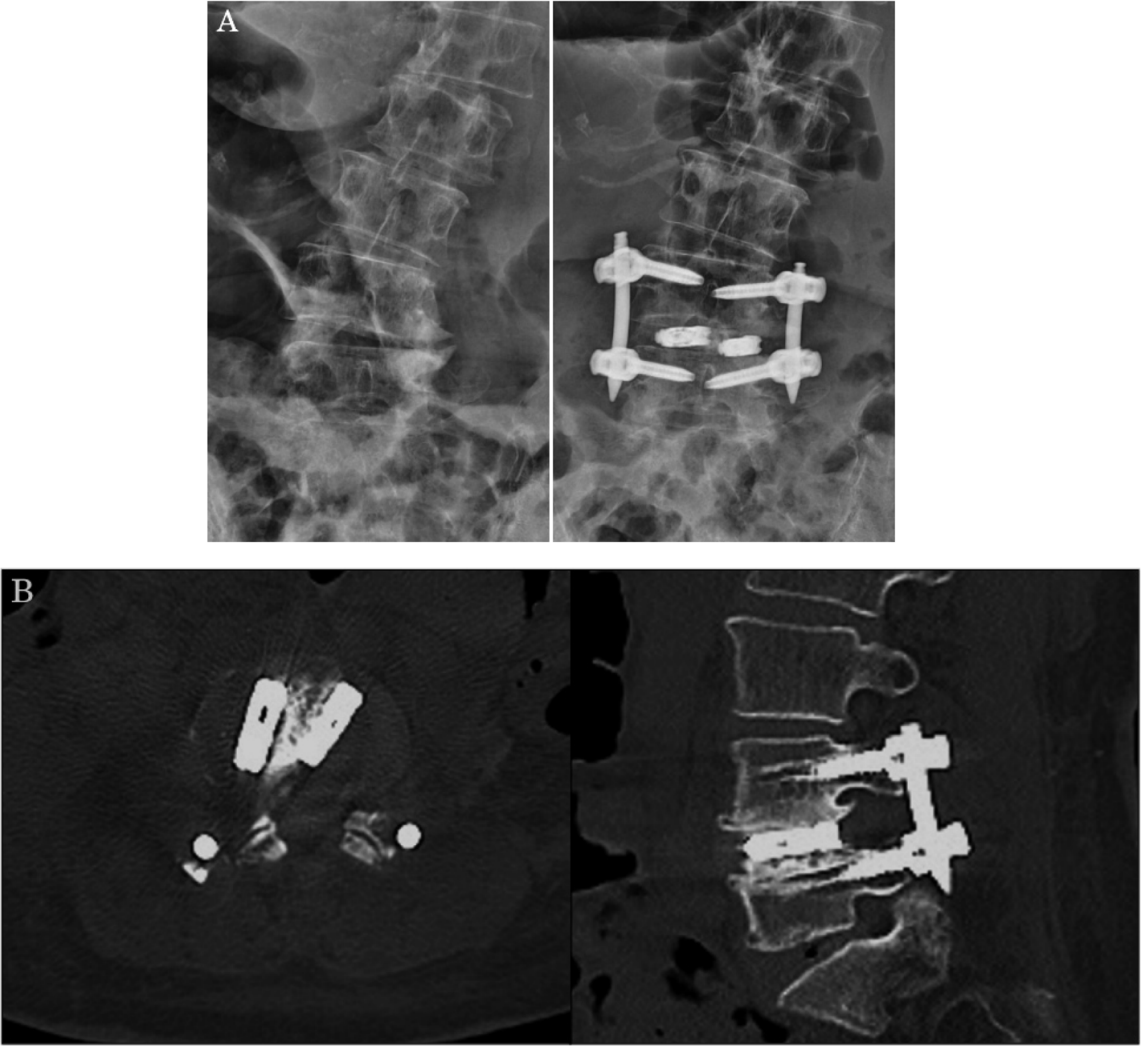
Insertion of two cages. a Anteroposterior X-ray films before and after surgery. b Computed tomography sagittal and axial images
